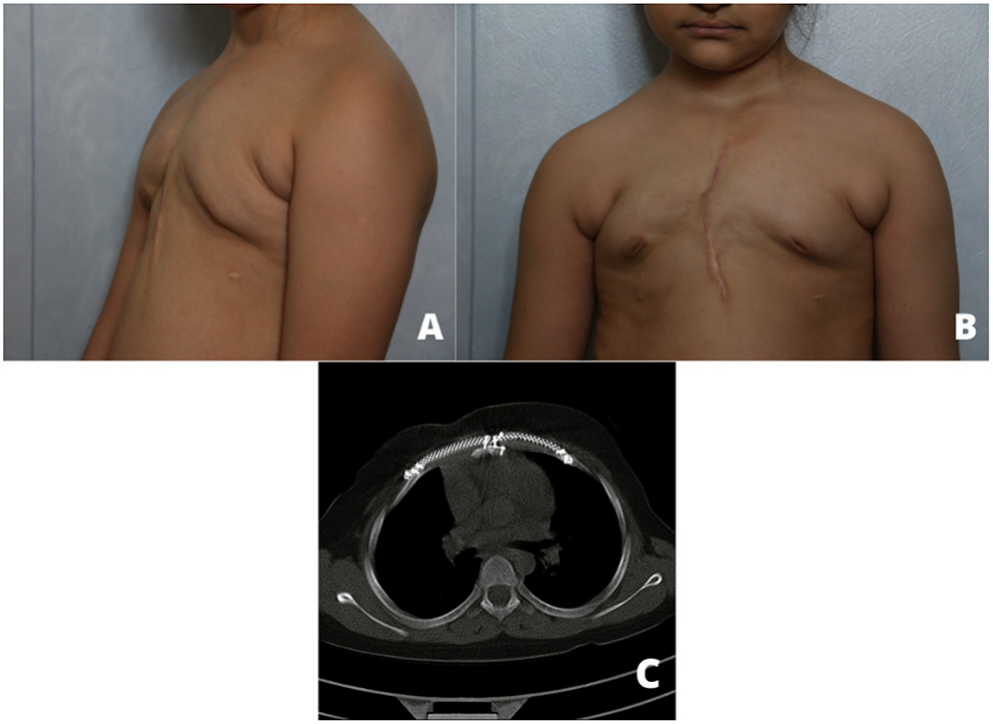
An anterior, lateral photograph (A,B) and CT scan (C) 16 months after the operation.
